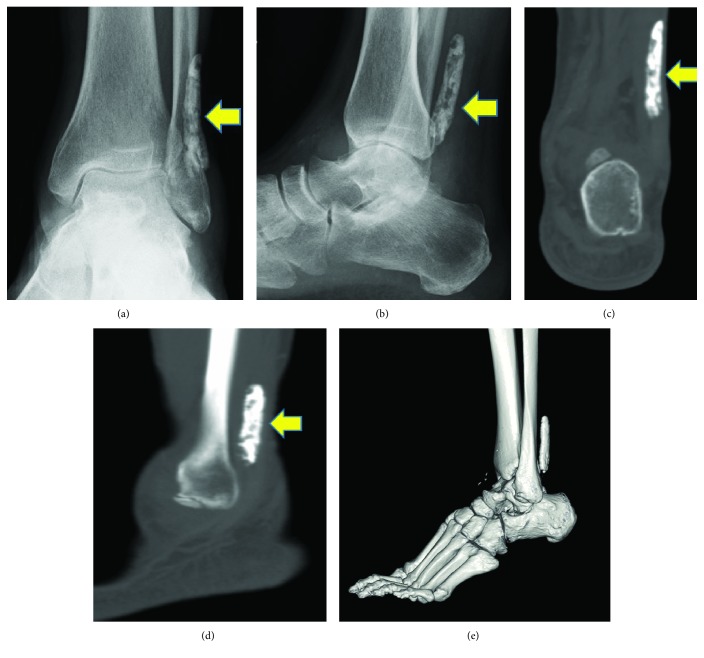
X-ray and CT showed a 1 × 5 cm elliptical opacification (yellow arrow) along the course of the peroneal tendon. (a) AP view of the X-ray image. (b) Lateral view of the X-ray image. (c) Coronal section of the CT image. (d) Axial section of the CT image. (e) 3D reconstruction of the CT image.
